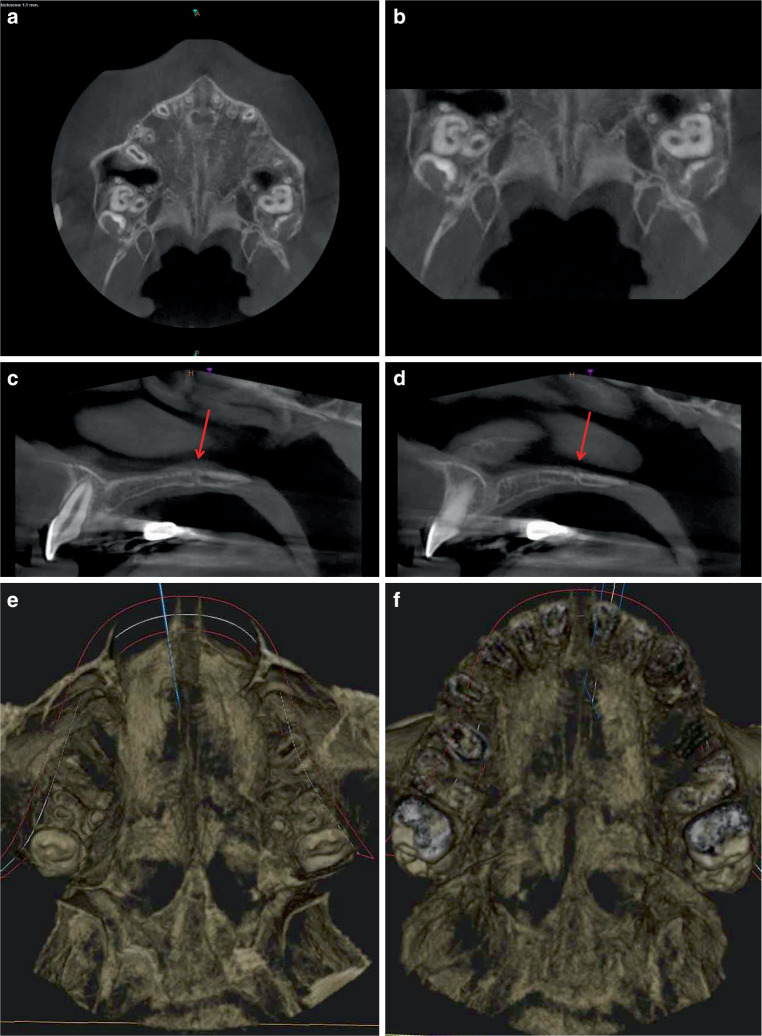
Horizontal (a, b) and sagittal slices (c, d) as well as 3D reconstructions with views from cranial and caudal (e, f) after combined rapid maxillary expansion/Delaire facemask (RME/DFM) treatment from a cone-beam computed tomography (CBCT) scan of a 10.10-year-old girl. a, b Slice (overview and detail) with open median and open anteriorly curved transverse palatal suture and partially open pterygopalatomaxillary sutures. c, d Both right and left lateral sagittal planes show a widely open transverse palatal suture (red arrows) with a straight, vertical course from cranial to caudal. e, f Parallel opening of the median palatal suture and wide open transverse palatal suture from cranial (e) and caudal (f). Clear quadripartition of the bony palate Horizontale (a, b) und sagittale Schichten (c, d) sowie 3‑D-Rekonstruktionen mit Ansichten von kranial und kaudal (e, f) nach kombinierter RME/DFM(„rapid maxillary expansion/Delaire facemask“)-Behandlung aus der DVT (digitale Volumentomographie) eines 10,10 Jahre alten Mädchens. a, b Schicht (Übersicht und Detailausschnitt) mit offener medianer und offener, nach anterior gewölbt verlaufender transversaler Gaumennaht sowie partiell offenen pterygopalatomaxillären Suturen, c, d Sowohl rechts- als auch linkslateral zeigt sich in der Sagittalebene eine weit geöffnete Sutura palatina transversa (*rote Pfeile*) mit geradem, senkrechten Verlauf von kranial nach kaudal, e, f Parallele Öffnung der Sutura palatina mediana und weit offene Sutura palatina transversa von kranial (e) und kaudal (f). Deutliche Vierteilung des knöchernen Gaumens
